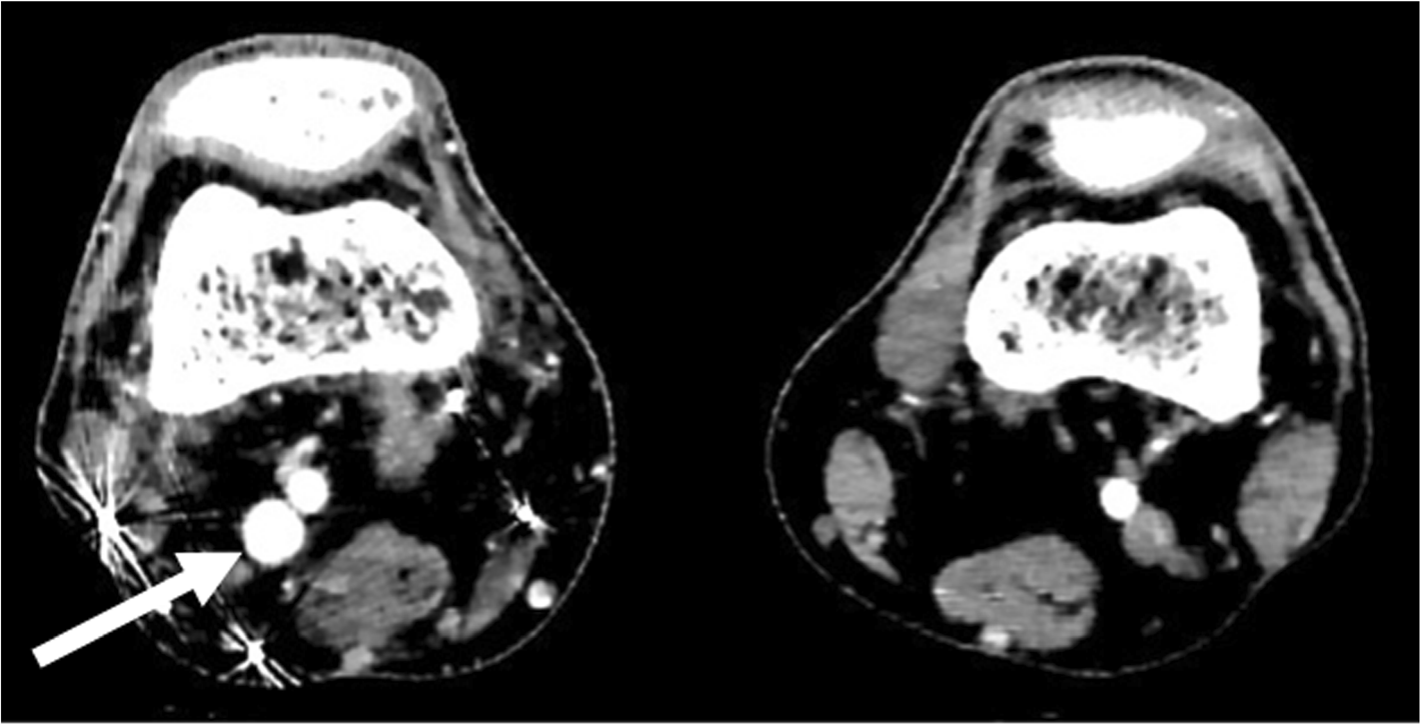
CTA showing early filling of right popliteal vein, indicating the presence of an AVF. Pellet artifacts in the posterior-lateral distal thigh
